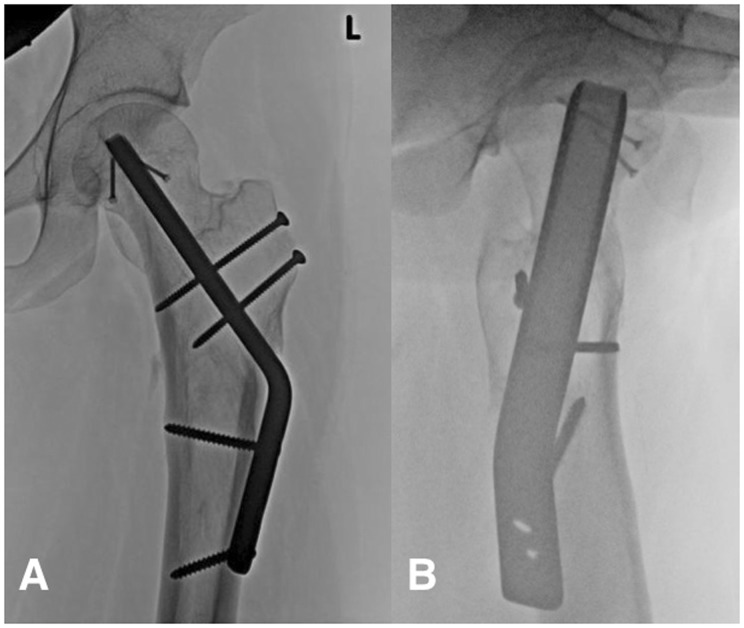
Two-year postoperative AP radiograph (A) and lateral (B) showing adequate fixation and consolidation of the trochanteric osteotomy without changes in the femoro-acetabular joint.
